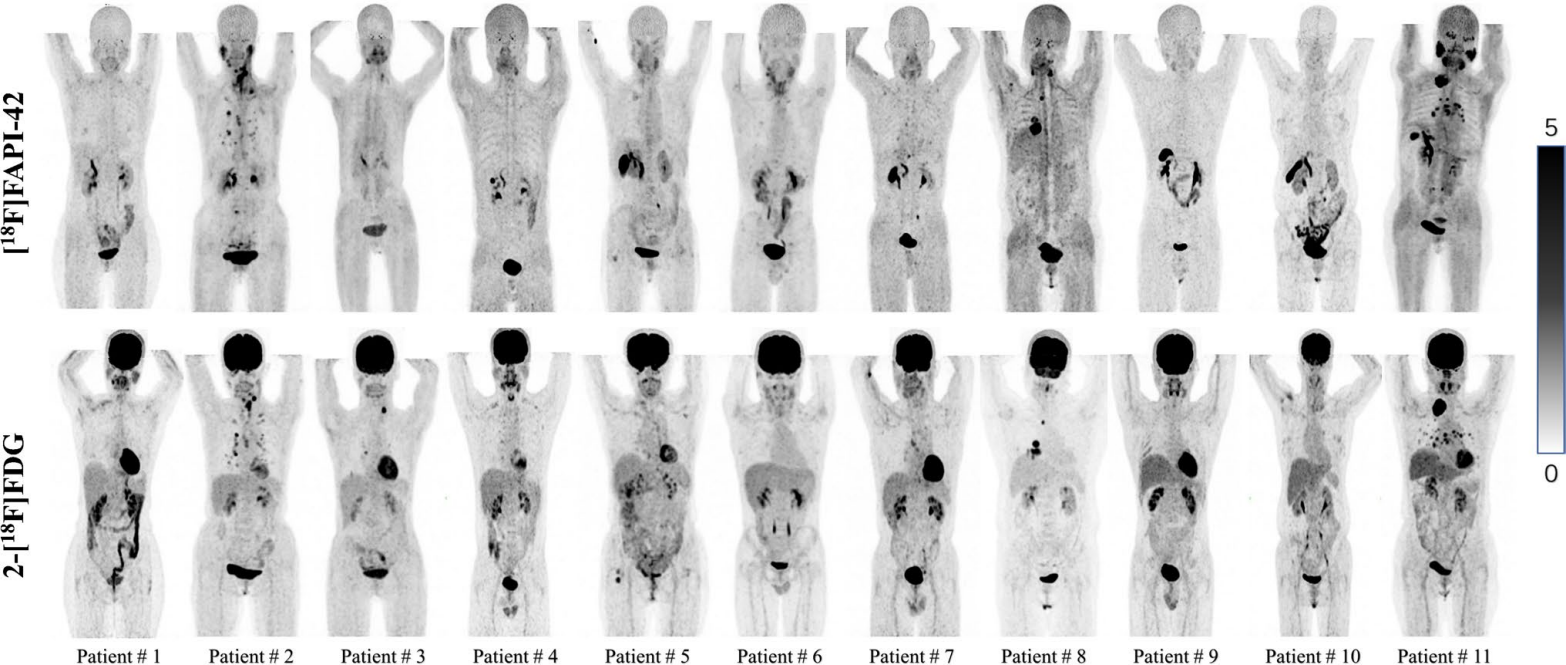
Maximum intensity projection images of all patients who underwent [^18^F]FAPI-42 and 2-[^18^F]FDG PET/CT
